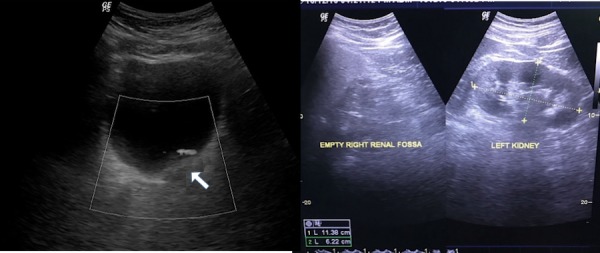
Ultrasonogram axial images In the left image, the white arrow demonstrates a bulky left-seminal vesicle whereas the right image shows an empty right renal fossa with compensatory hypertrophy of the left kidney. No ectopic kidney was found.
